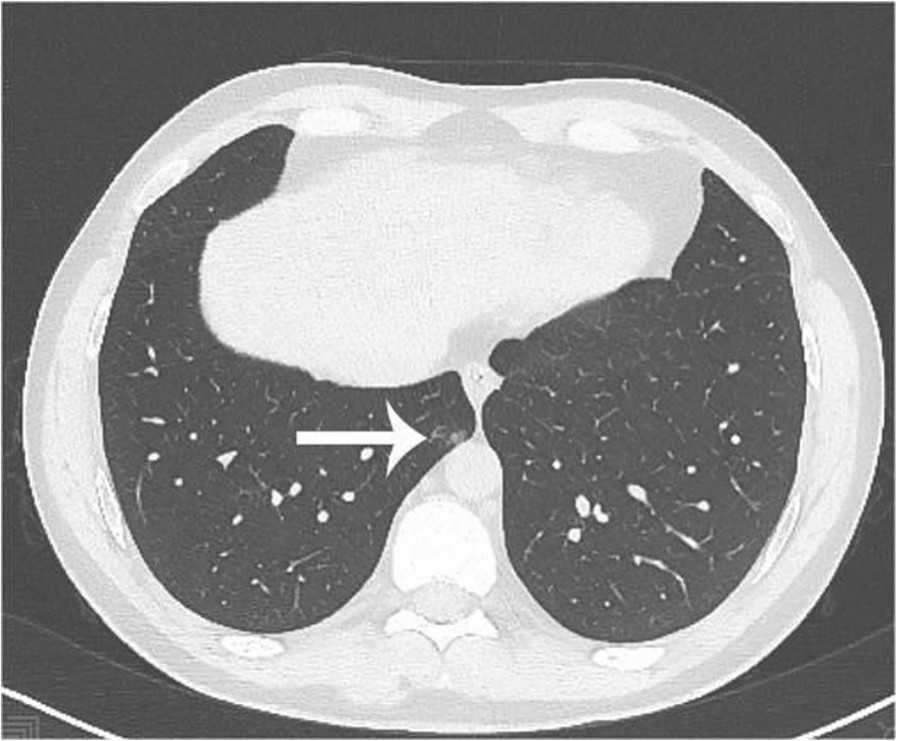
Positive CT finding in RT-PCR negative case with a history of contact. In this 34-year-old male patient with negative RT-PCR results and with COVID-19 positive family members. There is only a single millimeter-sized lesion in ground-glass opacity, subpleurally located in the right lobe medial lower lobe, significant in terms of involvement on CT (arrow)
